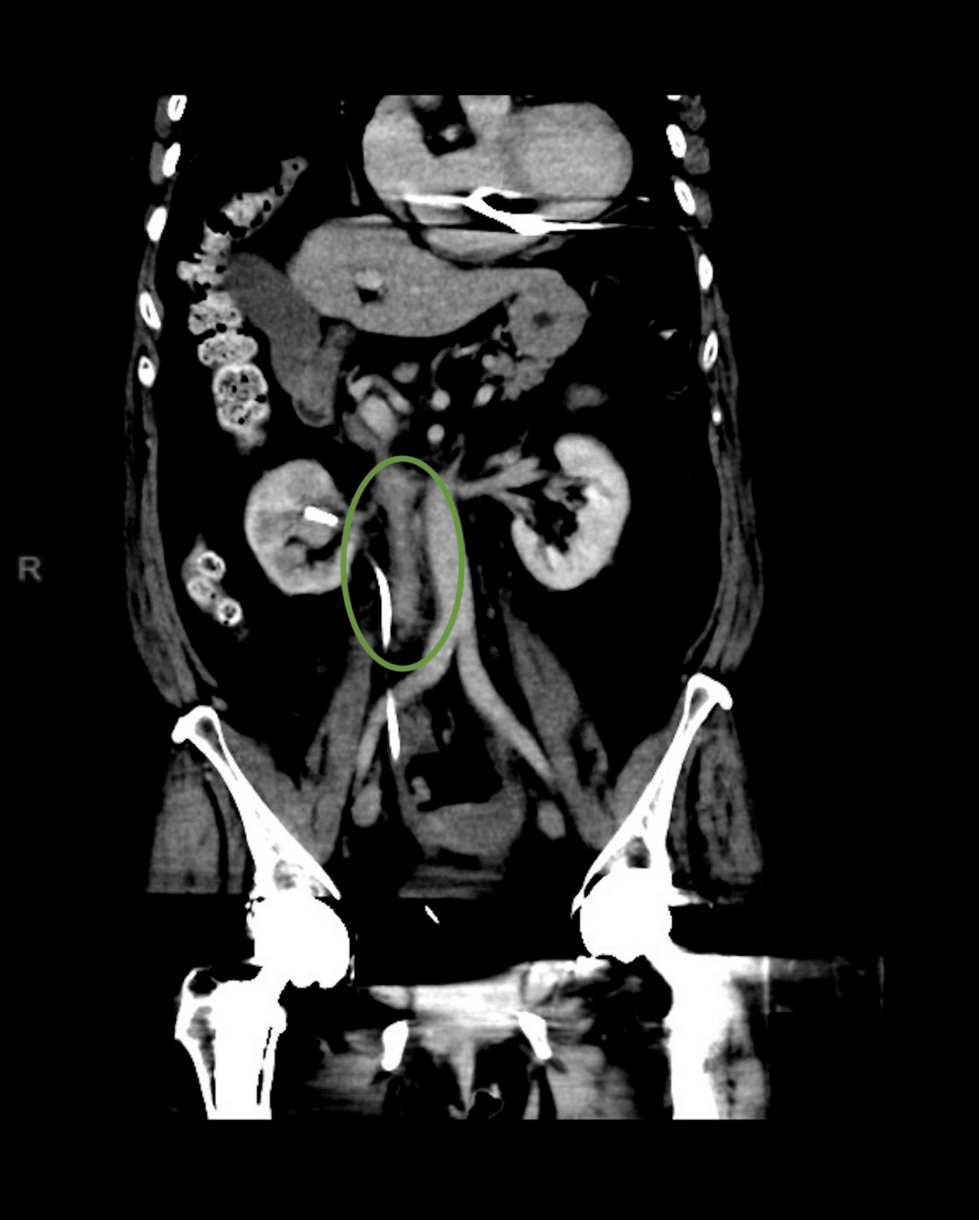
CT of the abdomen and pelvis (coronal view) showing an ill-defined soft tissue mass, predominantly right-sided, extending from the renal hilum to the aortic bifurcation CT: computed tomography
